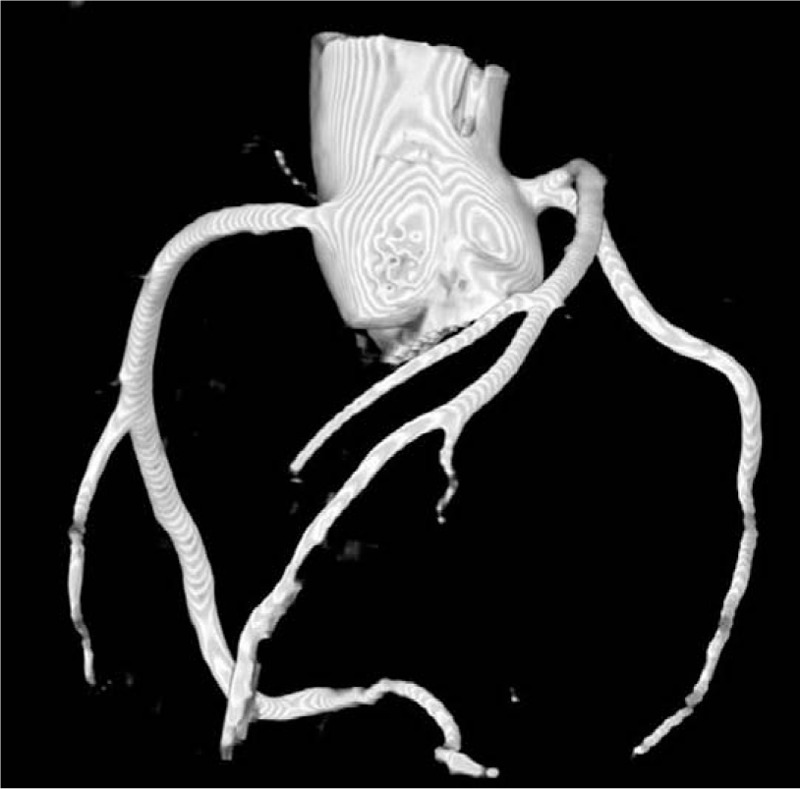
Three-dimensional reconstruction of coronary postmortem computed tomography angiography shows no abnormalities in the various major branches.
